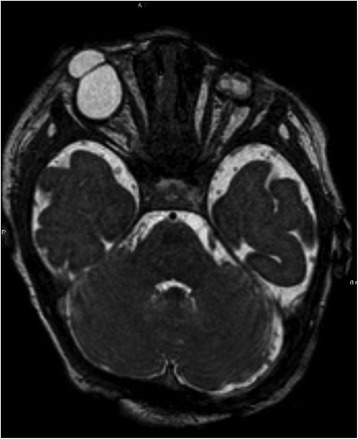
CT revealed microphthalmos into left ocular globe and a structure like-coloboma into right ocular globe
